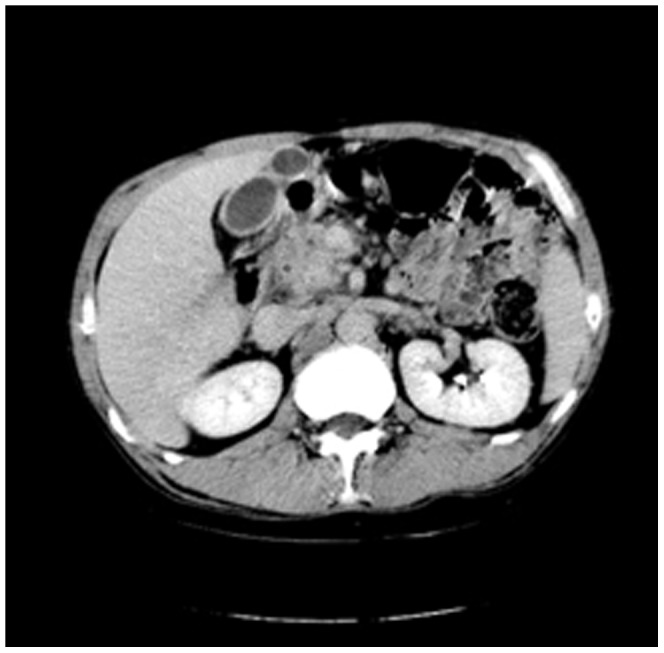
Enhanced CT showing a capsule-like rim.
